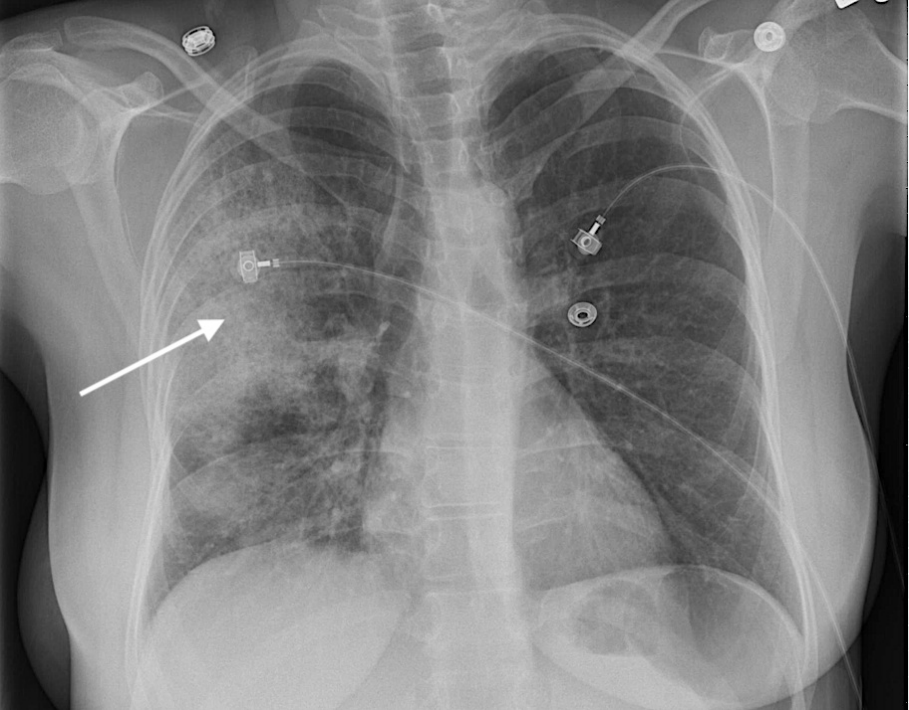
Patient's initial chest X-ray with arrow depicting right upper lobe pneumonia.
